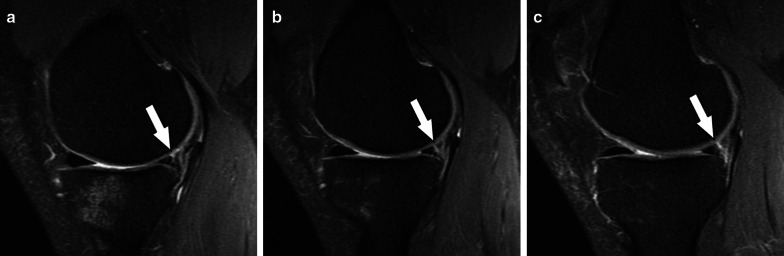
Type 2 meniscal ramp lesion MRI Case. Sequential sagittal T2-weighted fat-suppressed MR images show peripheral partial tear of posterior horn of medial meniscus (arrows) affecting the femoral articular surface, along with meniscocapsular ligament tear
